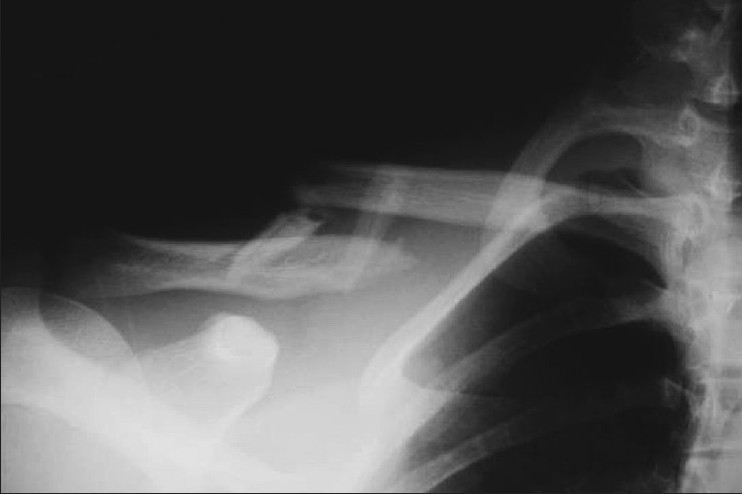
The radiograph showing 100% displacement and more than 2 cm shortening of mid-shaft clavicle fracture
